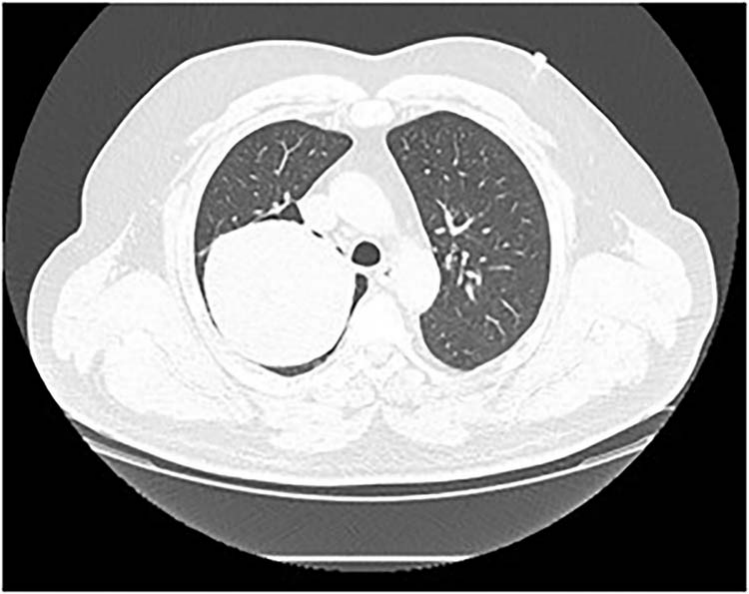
Chest CT scan. A well-defined solid nodule of 10 cm in diameter in the right upper lobe.
